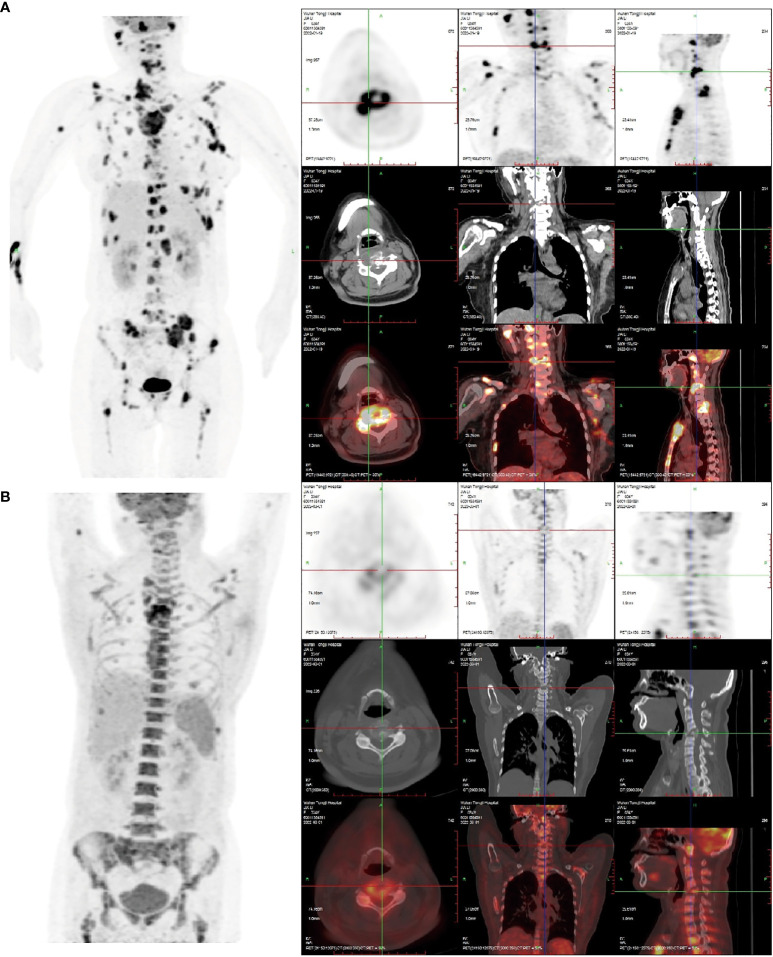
(A) The 18F-FDG PET/CT image on January 20, 2022: Right frontal bone, jaw bone, occipital slope, right humerus, sternum, bilateral clavicles, bilateral shoulder blades, bilateralmultiple ribs, extensive cervical/thoracolumbar vertebrae appendages, pelvic bones, and bilateralfemurs were involved. Some lesions were associated with soft tissue masses, with significantlyincreased radiotracer uptake. The size of the soft tissue masses in the sternum region wasapproximately 5.5 cm×2.9 cm×3.8 cm, and the SUVmax was 16.8. Increased and enlargedlymph nodes were seen in the right parotid gland region, right supraclavicular region, and leftarmpit, with increased radiation uptake. The largest lymph node was in the left armpit(approximately 1.6 cm × 1.4 cm in size, SUVmax 15.6.). (B) The 18F-FDG PET/CT image of thepatient after receiving two courses of chemotherapy on March 19, 2022. Compared with the (A) image, in the (B) image shown, there was a significant reduction or disappearance of radioactivityuptake in multiple bones and multiple lymph nodes, including the virtual disappearance of thesoft tissue mass in the sternal region, and a reduction of radioactivity uptake in the adjacentsternum compared with the previous one (SUVmax: 10.6). The left axillary lymph node lesiondisappeared without significant radioactivity uptake. Furthermore, bone fractures exist, and thebone marrow metabolic activity is increased (likely due to the effect of colony-stimulatingfactors).
